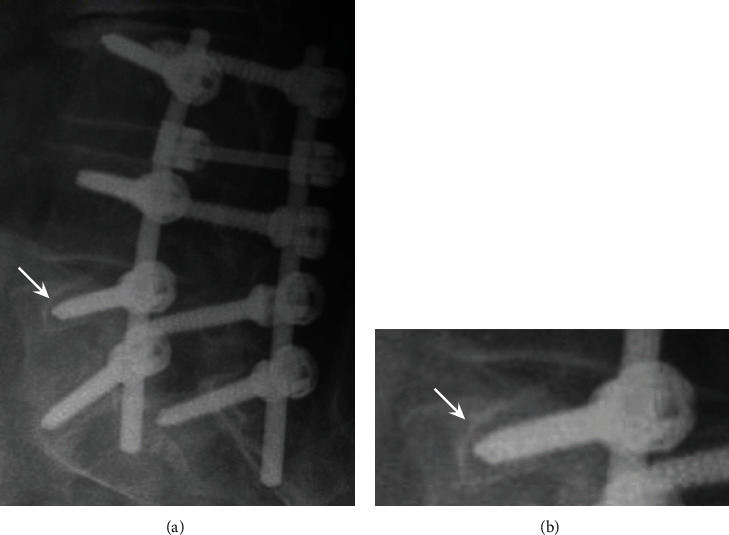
(a, b) Radiography of the lumbar spine with resorption of bone in the vertebral body of L5 leading to hardware loosening.
